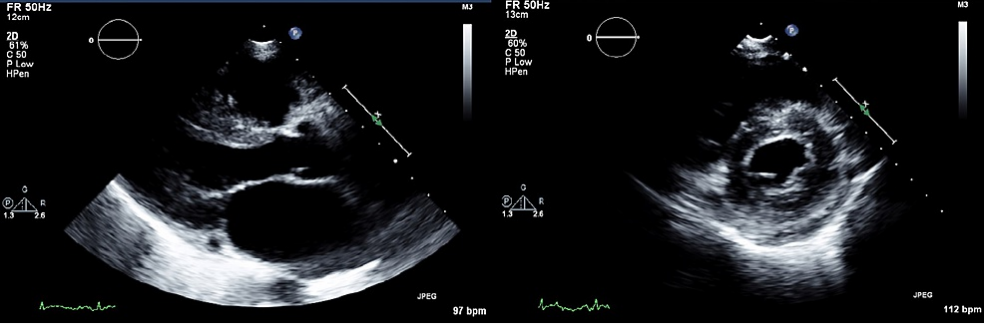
Left side: Parasternal long-axis view of the echocardiogram at baseline showing non-dilated left ventricle; right side: parasternal short-axis view at papillary muscle level showing non-dilated left ventricle (April 2021).
